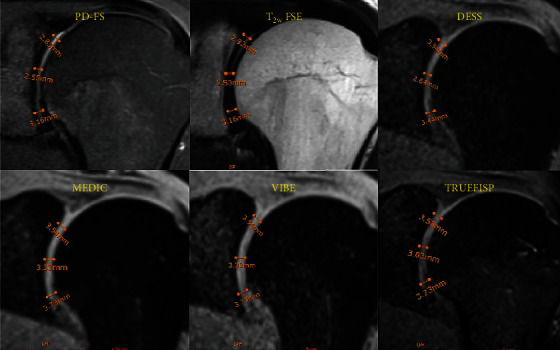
Conventional two-dimensional (2D) and three-dimensional (3D) MRI images of shoulder joint in a 51-year-old male. Glenohumeral space was measured in superior, middle, and inferior regions of the shoulder joint, and then, the mean glenohumeral space was calculated.
